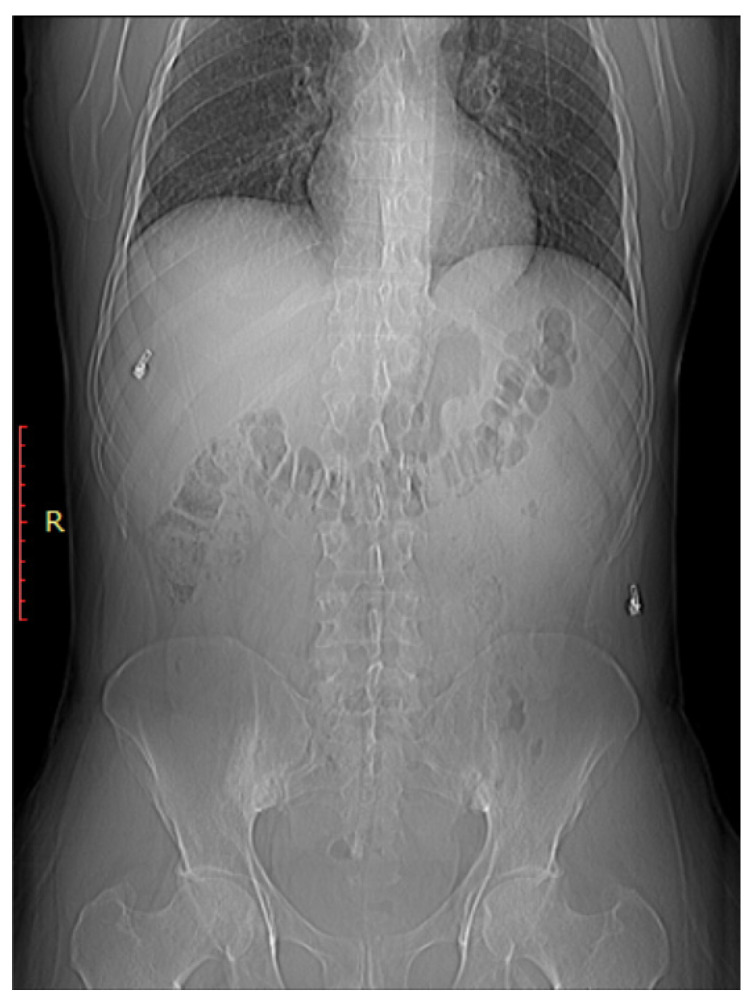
Bilateral sacroiliitis. X-ray for a 41-year-old male presenting with Rt sciatica showing irregularity and sclerosis of both sacroiliac joints is more evident on the right.
